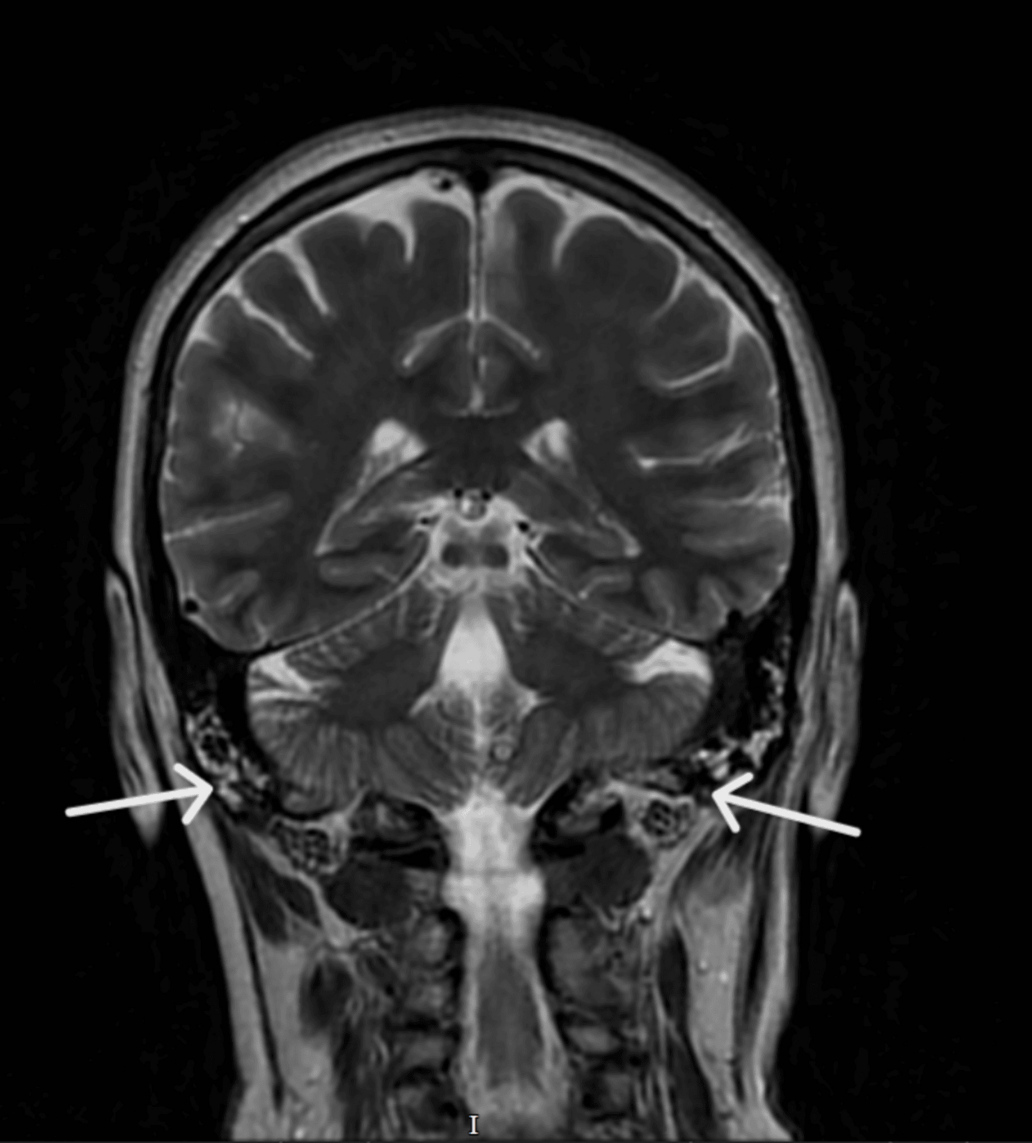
MRI brain (T2 coronal): Bilateral petromastoid inflammation noted, more prominent on the left.
